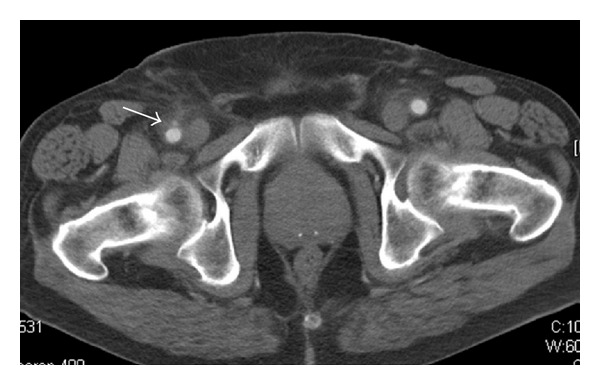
Small aneurysma spurium of the right common femoral artery (arrow) which was detected in CT imaging three days after percutaneous EVAR. The aneurysm could be treated successfully with compression.
